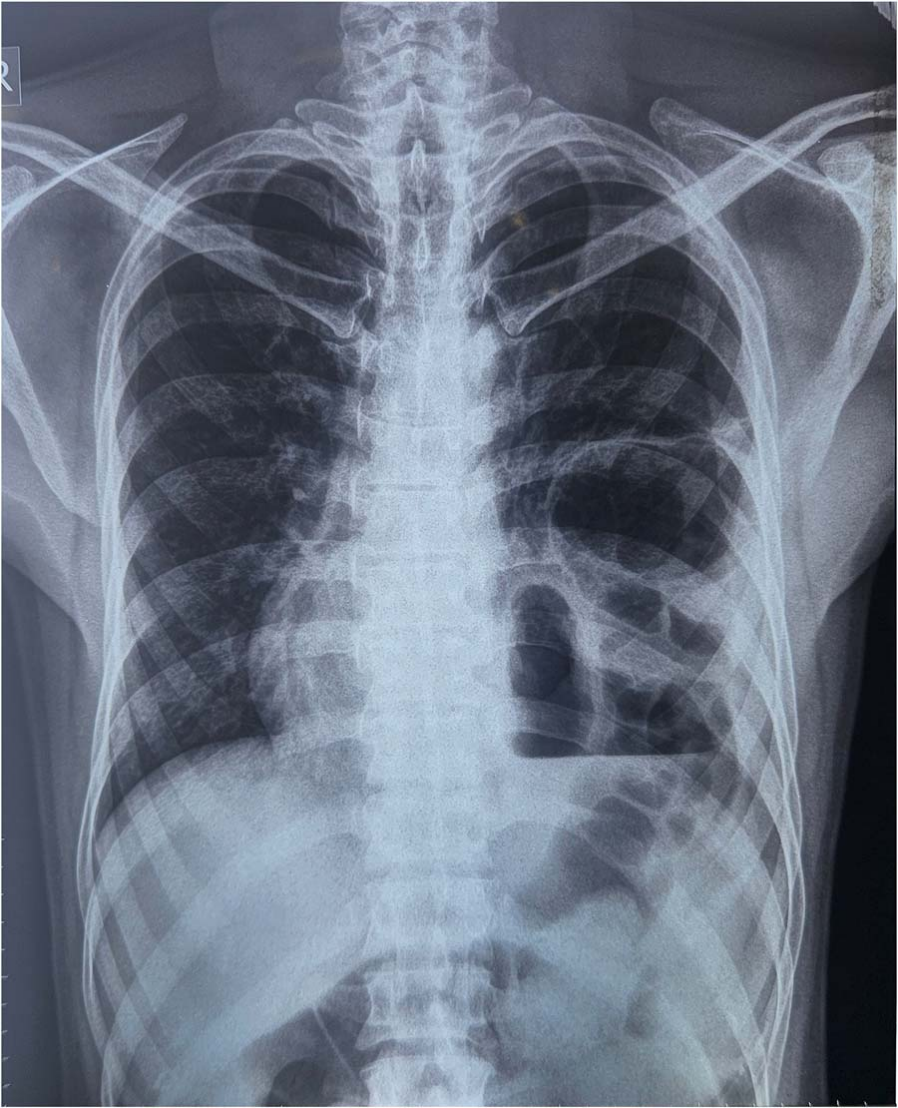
X-ray s/o air-fluid level in left hemithorax.
